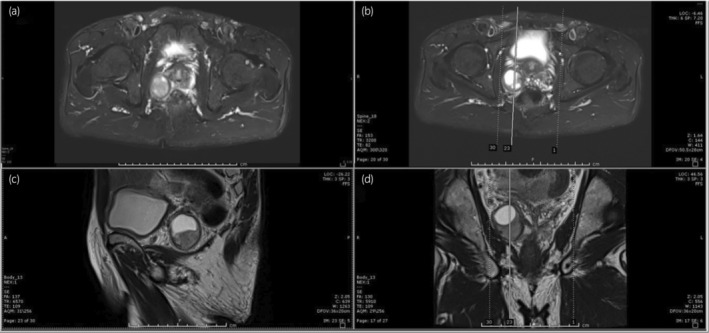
Coronal (a) and axial (b) T2‐weighted MRI of PI‐RADS 4 adenocarcinoma. Coronal (c) and axial (d) T2‐weighted MRI of periprostatic cystic lesion.
